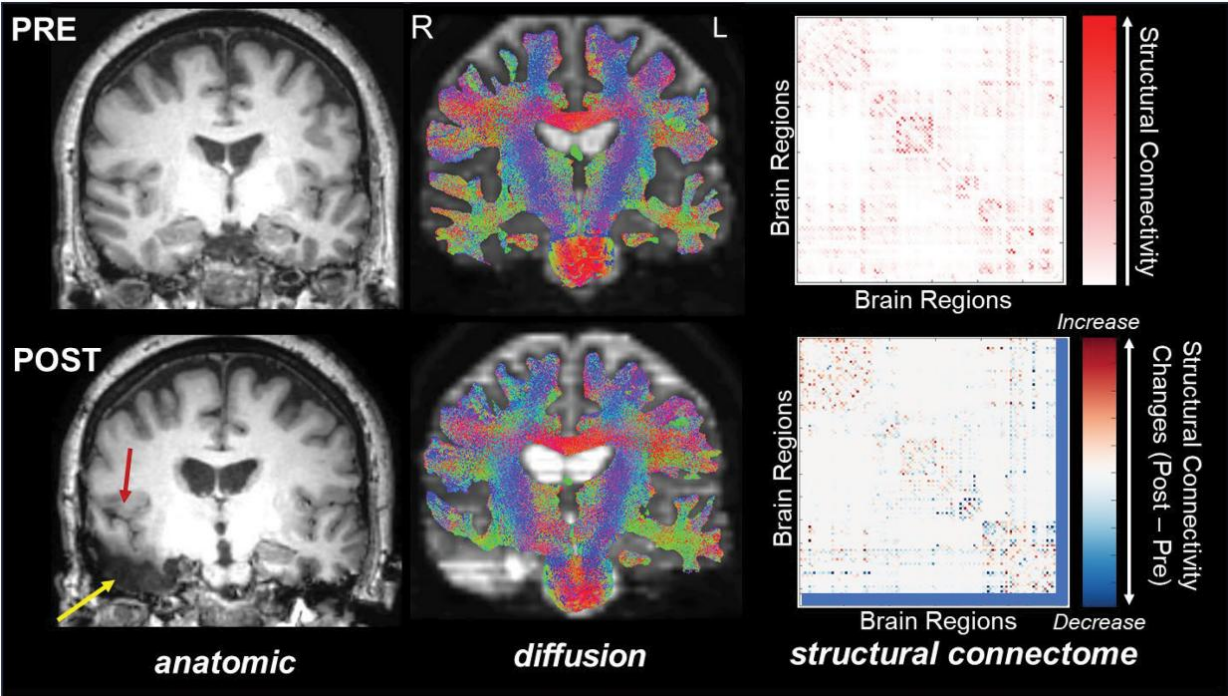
Anatomic MRI (*left*), diffusion tractography (*middle*) and structural connectomes (*right*) acquired before and after right temporal lobectomy in a patient with unilateral temporal lobe epilepsy. Anatomical images highlight the resection cavity (yellow arrow) and brain shift (red arrow) after surgery. The lower structural connectome highlights both connectivity increases and decreases from pre- to post-surgery. Blue bars indicate removal of resected regions from the connectome. L = left; R = right.
